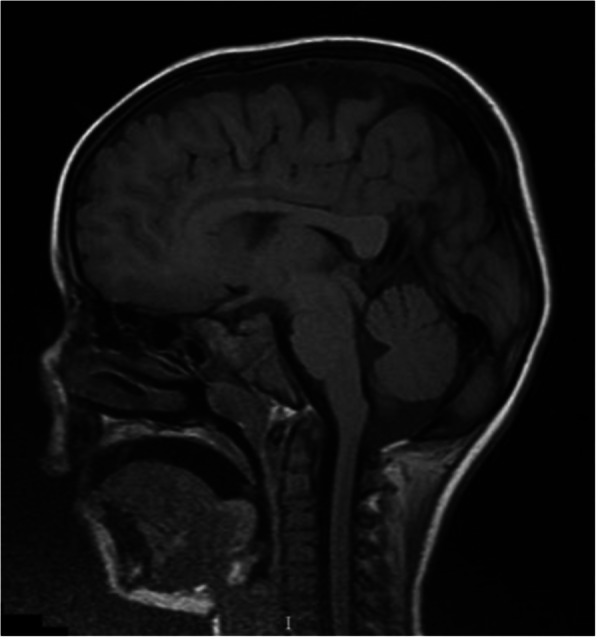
MRI DWI: lesion of the splenium of corpus callosum (sagittal section)
